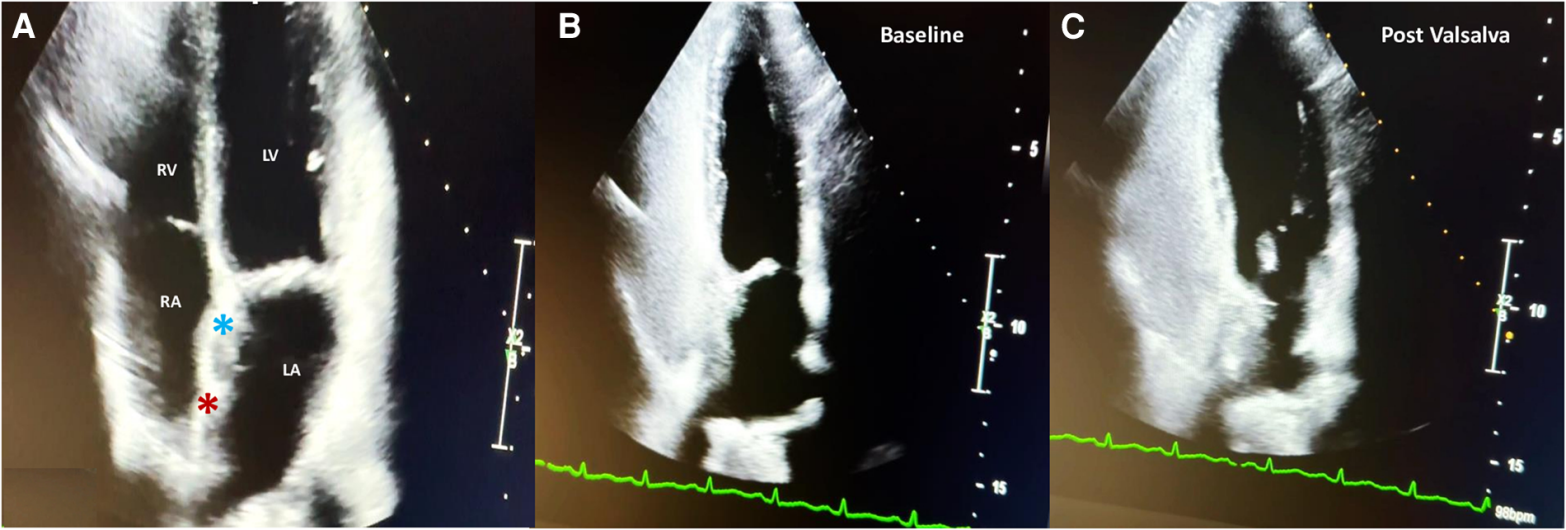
Two-year follow-up 2D TTE color Doppler in the apical four-chamber view showing the correct interaction between the UNI 33/33-mm device (light blue asterisk) and the Figulla Flex II 16/18-mm device (red asterisk) (A); contrast 2D TTE confirming abolition of the residual shunt at baseline (B) and after the Valsalva maneuver (C).
